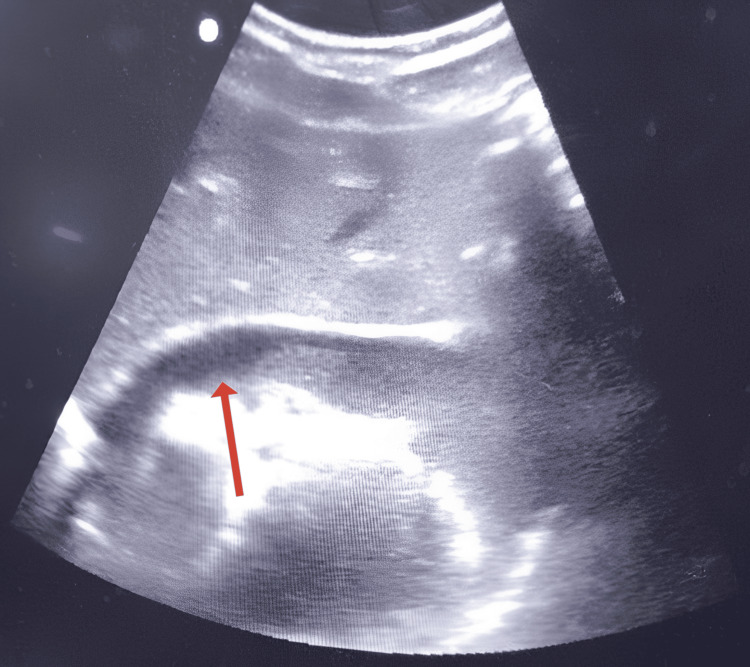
EFAST image showing a significant anechoic pericardial effusion, raising suspicion for cardiac tamponade EFAST, extended focused assessment with sonography in trauma
